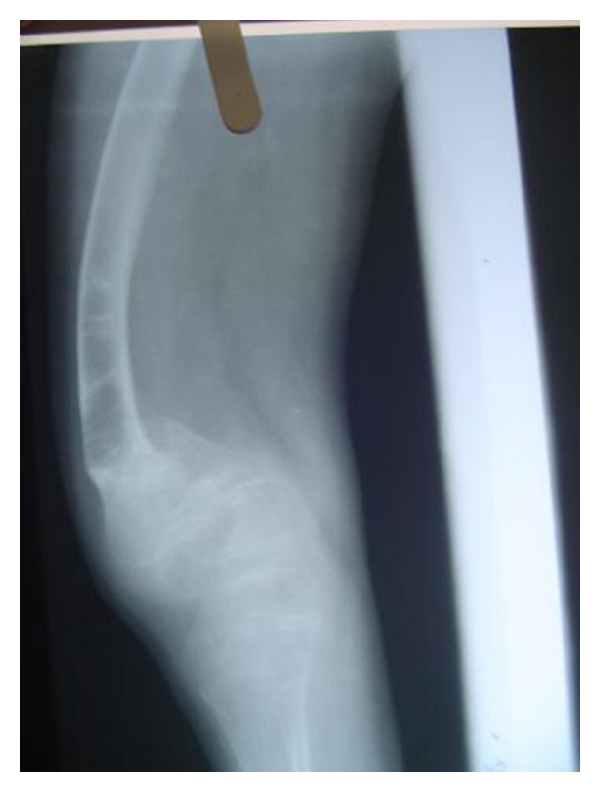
Radiograph of the lower limbs showing bowing of the femur, with widening of the metaphases.
